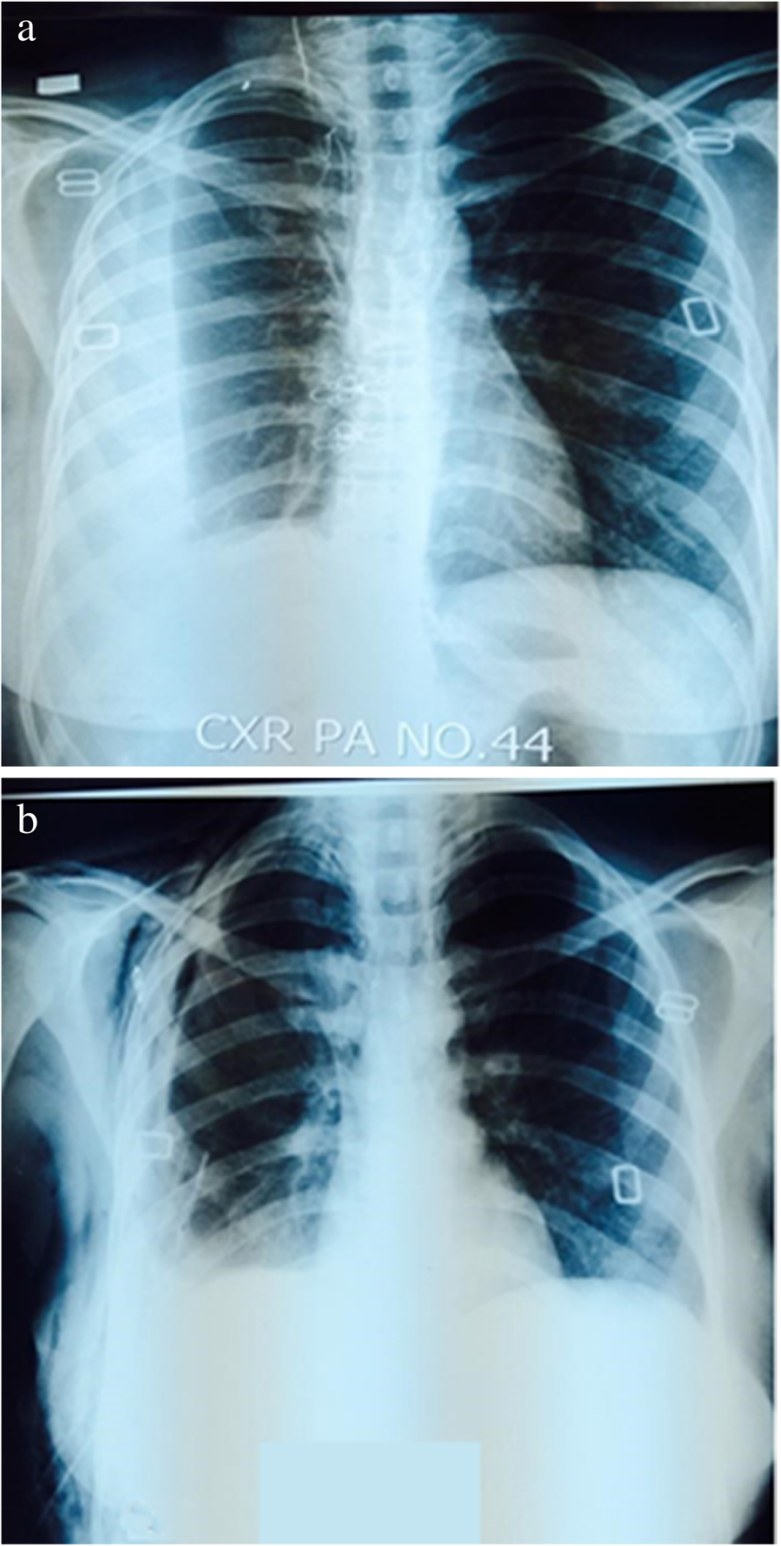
Comparison of chest X-rays before and after medical Thoracoscopy (a) chest x-ray before thoracoscopy showing loculated pleural effusion (b) chest x-ray after thoracoscopy showing complete resolution of multiloculated and organized empyema
